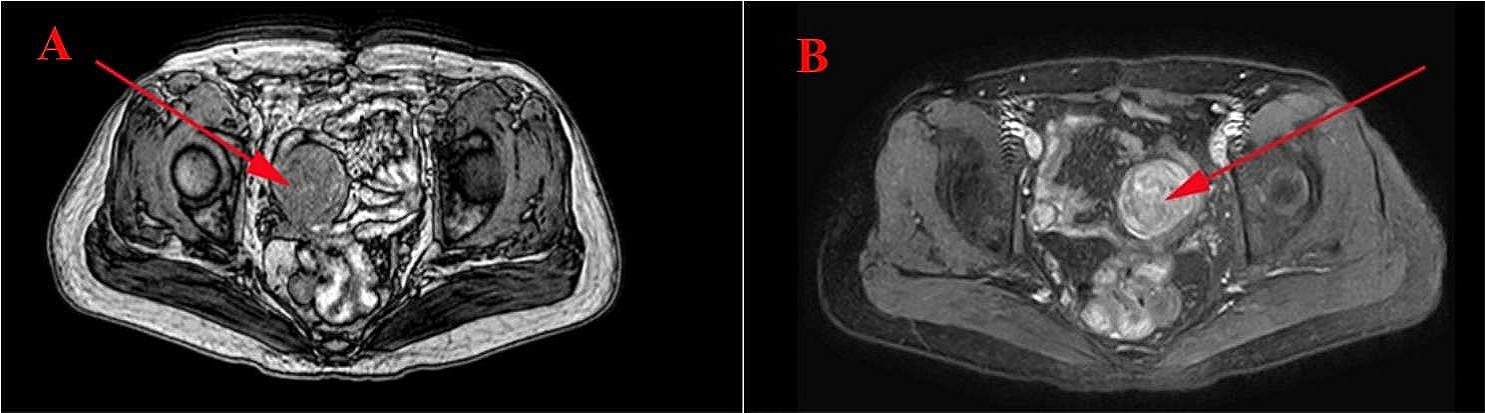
A cluster of abnormal signal lesions is seen in the left pelvic area, with a maximum cross-section of approximately 5.0 × 4.2 cm. The boundary is still clear, and the signal is uneven. It is iso-hypointense on T1WI and slightly hyperintense on T2WI. (A) DWI is hyperintense (A). Enhancement is uneven, and the boundary between the lesion and the left adnexa is unclear. (B). The red arrow indicates the location of the tumor
